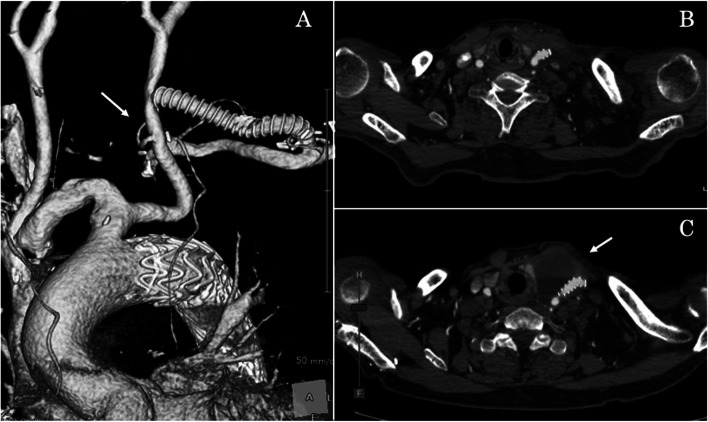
Postoperative computed tomography findings. A/B The arch aneurysm is excluded. The left subclavian artery-left common carotid artery bypass and the left vertebral artery (arrow) are patent. C The arrow indicates neck swelling caused by a chyle leak. D The picture shows puncture fluid of neck swelling caused by a chyle leak
